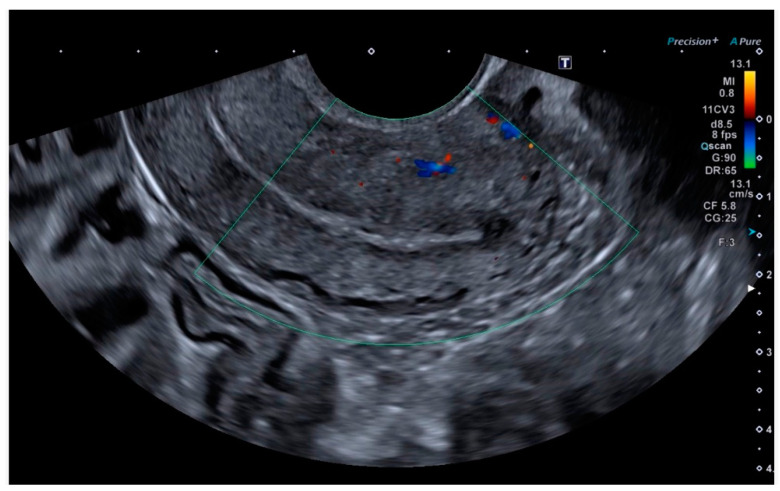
Absence of colour Doppler uptake.
